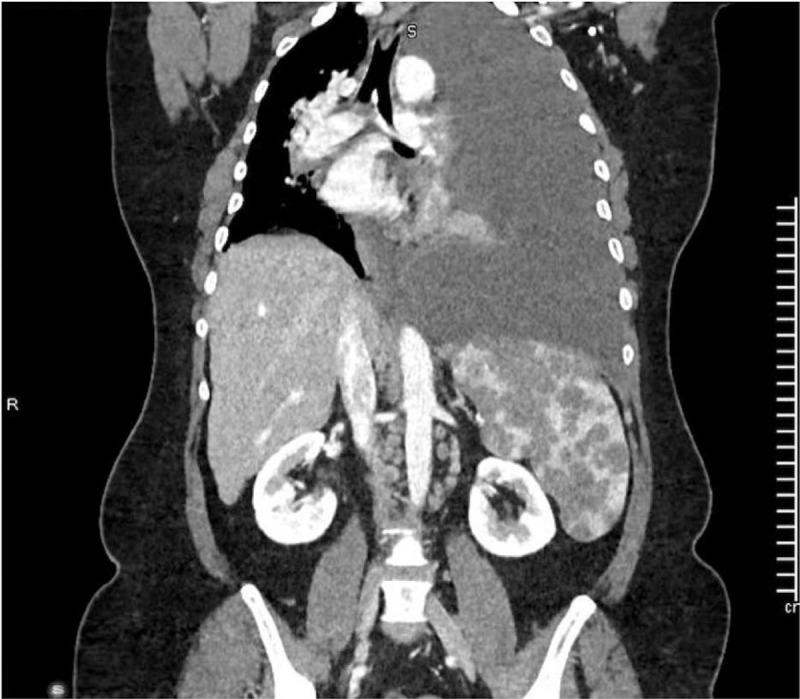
Chest and abdomen CT scan (coronal view) showed massive left sided pleural effusion, mediastinal shifting, multiple splenic hypo-attenuated nodular lesions along with multiple abdominal paraaortic lymph nodes. CT = computed tomography.
